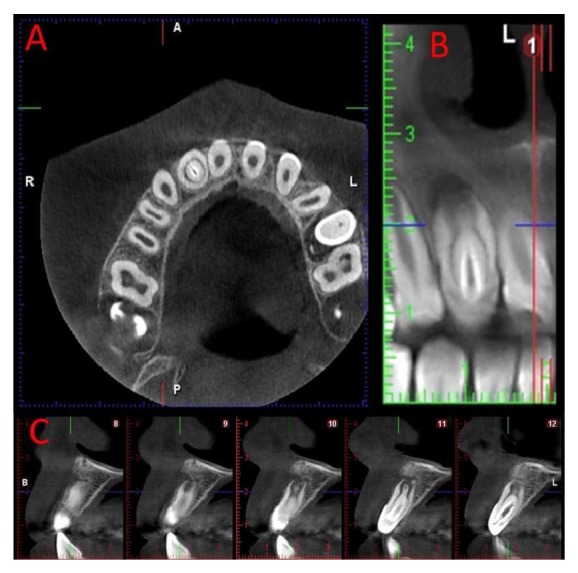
Three-dimensional image taken with CBCT.
